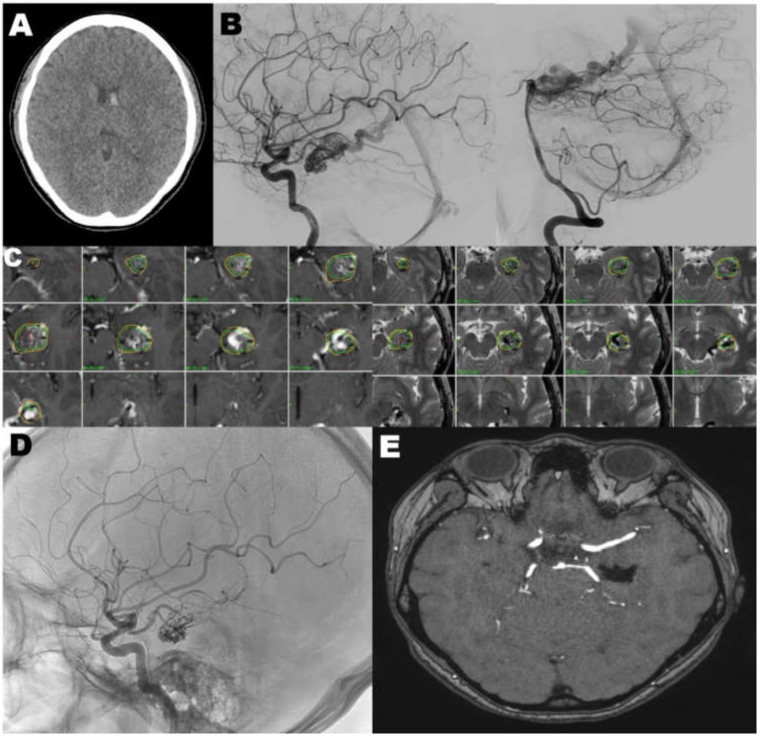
(A) The non-contrast enhanced computed tomography showed an acute intraventricular hemorrhage. (B) The digital subtraction angiography confirmed a Spetzler-Martin grade III AVM in the left temporal lobe with feeders from the left anterior choroidal artery and left posterior cerebral artery with venous ectasia, draining to the left basal vein of Rosenthal. (C) Gamma knife radiosurgery (3.30 cm^3^, 18 Gy to 50% of the isodense line). (D) The final angiogram after Onxy embolization showed near complete occlusion of the AVM nidus with multiple intranidal aneurysms. (E) The last follow up magnetic resonance imaging (24 months after GKRS) revealed neither residual nidus nor flow void.
